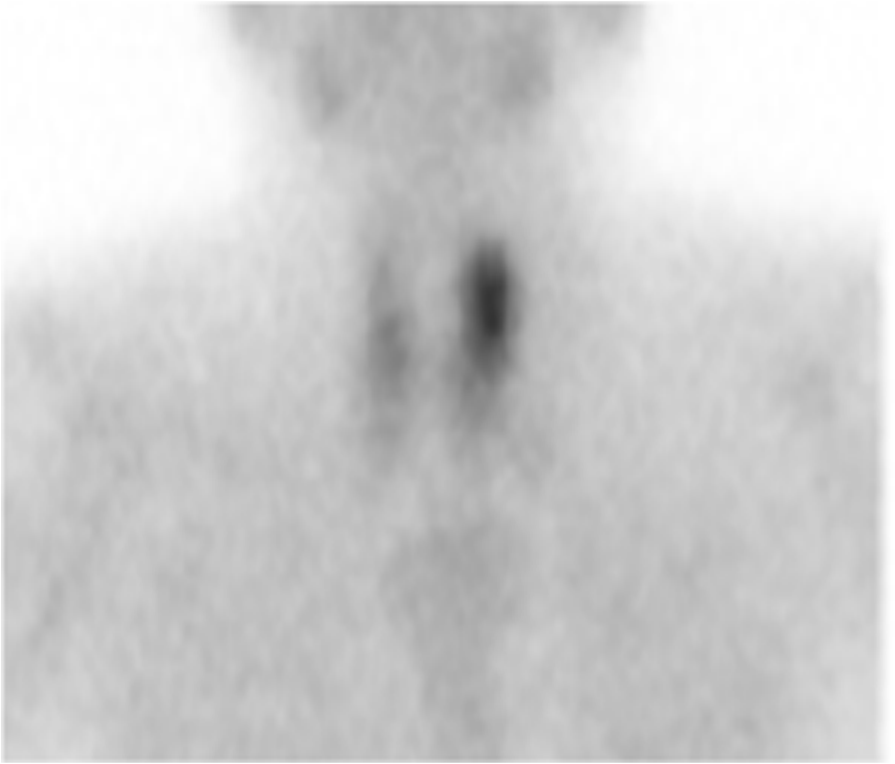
^99m^Tc-hexakis-2-methoxyisobutylisonitrile scintigraphy (Early phase))
